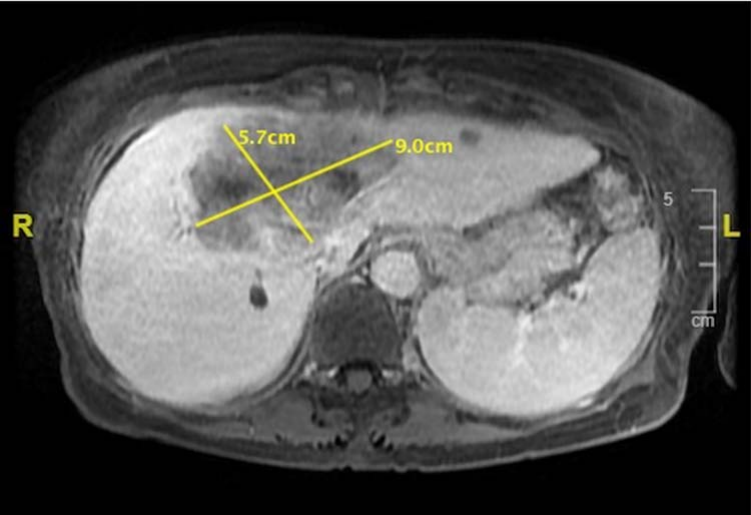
Axial MRI Liver demonstrating response to brachytherapy.
